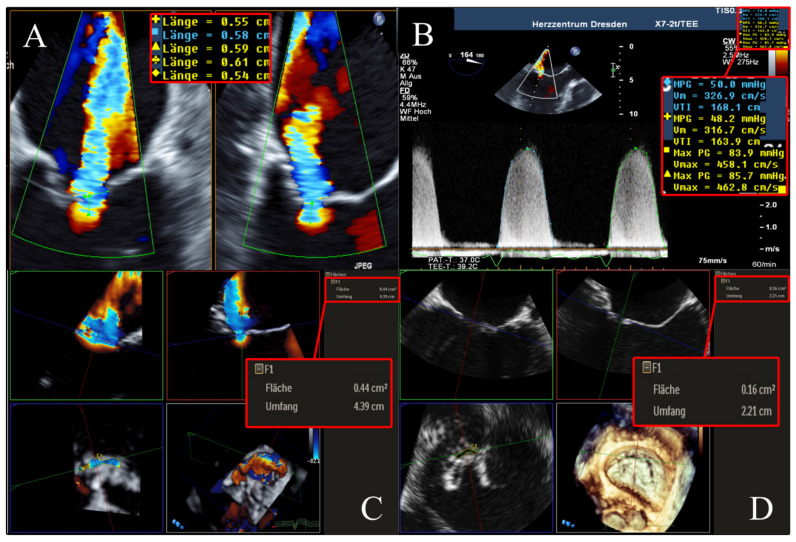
Example of a 51-year-old, male patient suffering from FMR due to non-ischemic dilated cardiomyopathy. (A,B) ERO quantified using 2D PISA method was 0.20 cm^2^, with a mean MR-Vmax of 460 cm/s. (C) 3D VCA derived by multiplanar reconstruction in a 3D color Doppler dataset was 0.44 cm^2^. (D) AROA derived by multiplanar reconstruction in a 3D Doppler dataset without color was 0.16 cm^2^. Abbreviations: FMR = functional mitral valve regurgitation; 2D = two-dimensional; PISA = proximal isovelocity surface area; CW = continuous wave; MR-Vmax = maximum mitral regurgitation velocity; 3D = three-dimensional; VCA = vena contracta area; AROA = anatomical regurgitation orifice area.
